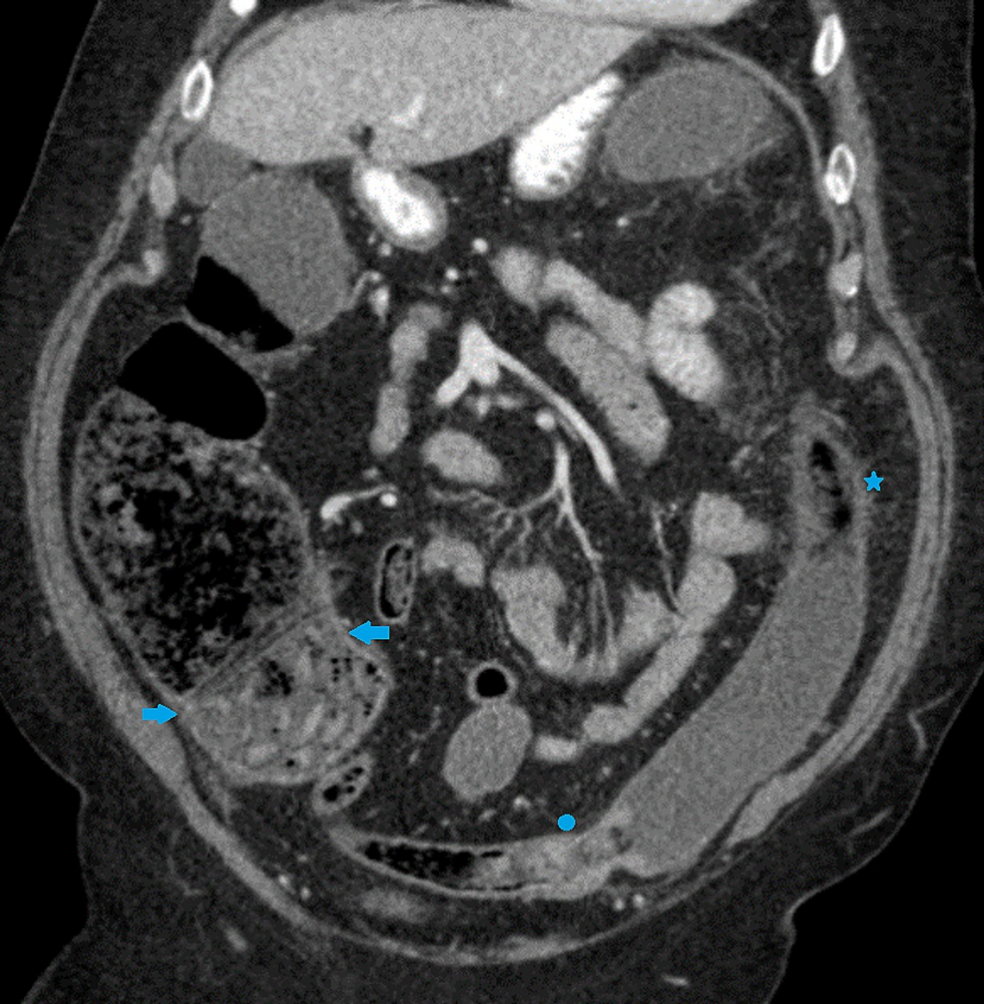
CT scan of the abdomen with contrast on admission demonstrating mild dilatation of the right colon with diffuse wall thickening and surrounding inflammation along the splenic flexure and descending colon CT: computed tomography
